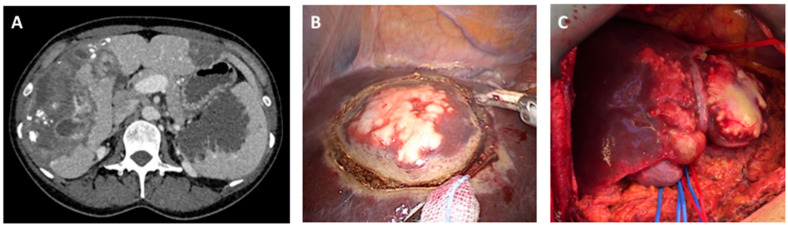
Pictorial summary of clinical and surgical aspects of alveolar echinococcosis (AE) in humans. (A) Computed tomography (CT) scan showing AE in the liver, spleen, and extraperitoneal areas. (B) An atypical laparoscopic resection of a lesion caused by AE from the liver dome. (C) AE in the left liver prior to left hemi-hepatectomy.
